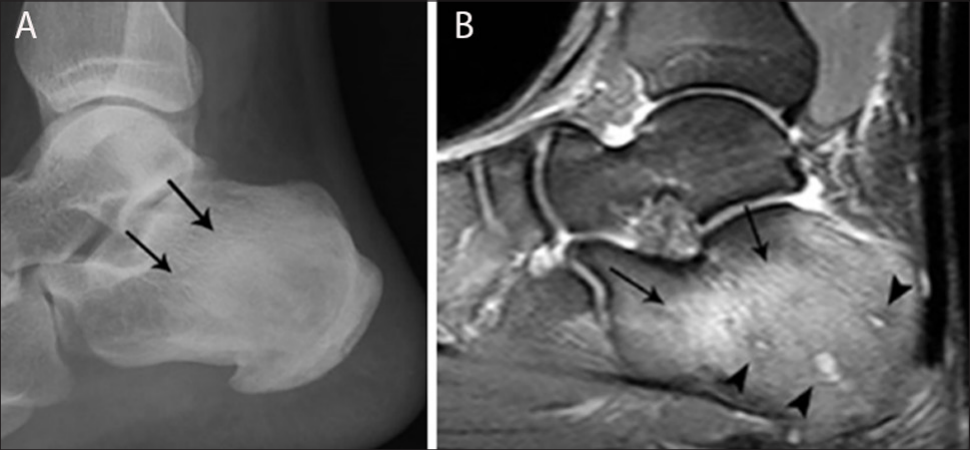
Chronic osteomyelitis of the calcaneus. Plain radiograph (a) and sagittal Fat–Sat T2–WI (b). Plain radiograph (a) shows inhomogeneous sclerosis (black arrows) in the calcaneus. Bone marrow edema (black arrows) is seen on MRI imaging (b), representing active infection. Note also the presence of small micro-abscesses (black arrowheads).
